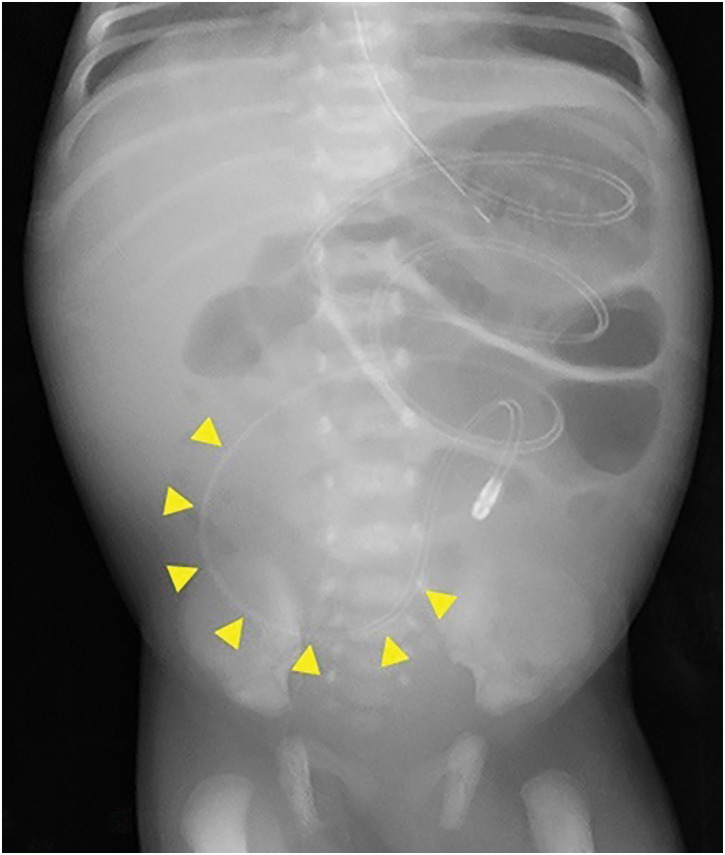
Abdominal radiography following the nasojejunal tube placement. There was no bowel dilatation proximal to the nasojejunal tube tip (arrowheads). However, radiography showed localized small bowel dilatation proximal to the left upper abdomen.
